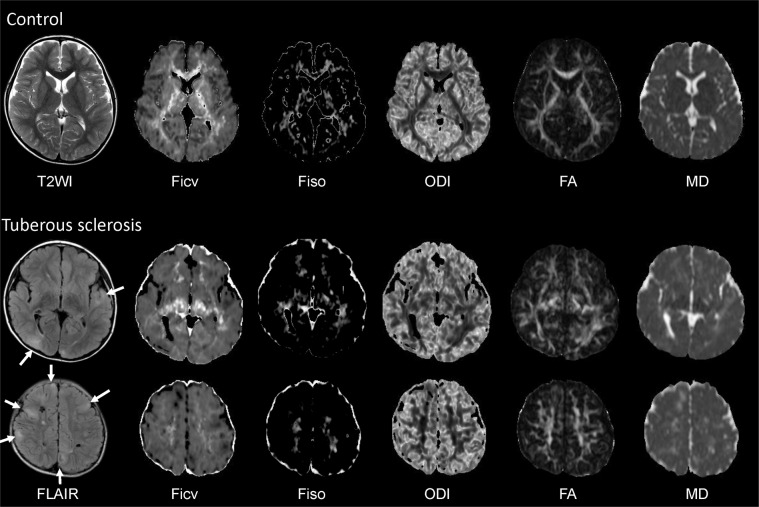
NODDI and diffusion tensor images of a control and a tuberous sclerosis (TS) case. Control (Upper row): 8-year-old female. TS case (Middle and lower row): 14-year-old female (Middle row: images at the level of the thalamus, Lower row: images at the level of the semioval center) For the control, the T2WI, and the Ficv, Fiso, ODI, FA and MD maps are shown respectively. The Ficv had higher values in the white matter, particularly in the projection fibers of the deep white matter. The intensity in the deep white matter had higher values of the Fiso as well. The ODI image indicated that the dispersion of the fiber orientation was smaller in the white matter especially in the deep white matter. The FA maps of the controls had higher values in the white matter, and the MD map indicated lower values in the white matter. For the TS case, the FLAIR images show cortical tubers (arrows) and white matter lesions as increased signal intensity. The Ficv map shows a lower intensity compared to the control both in the deep white matter (projection area) and the subcortical white matter (association area). On the Fiso map, the deep white matter has rather higher intensity, while the subcortical white matter is very low. On the ODI and FA maps, the control and TS case look very similar qualitatively. On the MD map, the cortical tubers and white matter lesions have a high signal intensity.
